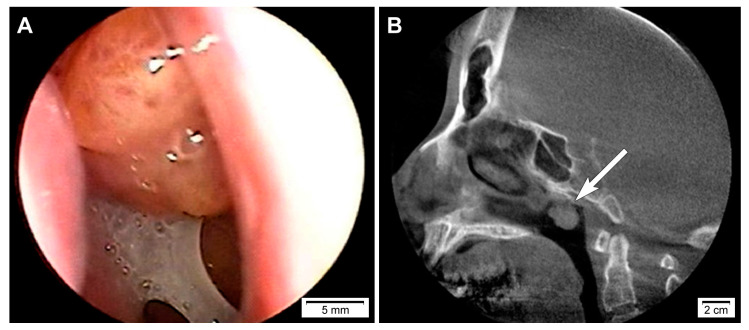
Imaging findings of biphasic thyroid-like low-grade nasopharyngeal papillary adenocarcinoma. (A) Nasopharyngoscopy and (B) computed tomography scan revealed a pedunculated mass arising from the roof of the nasopharynx (long white arrow).
